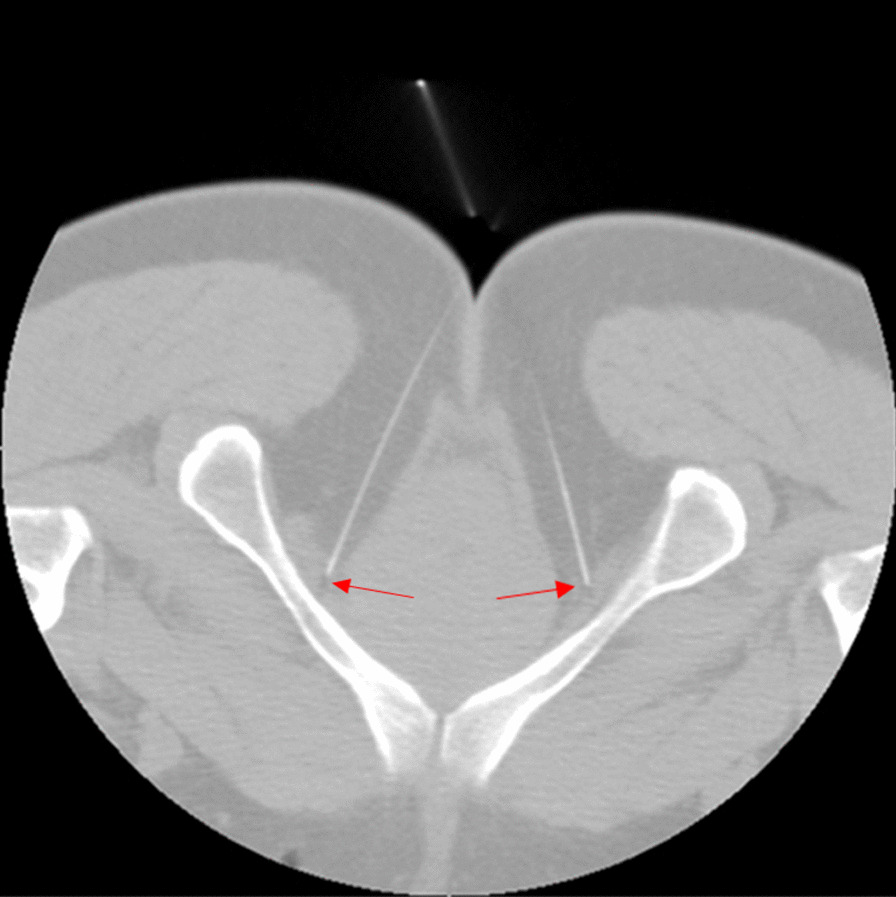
Transverse computed tomography (CT) scan of the coccygeal apex: A transverse CT image of the coccygeal apex shows the tips of needles inserted at the lower sacral point in the ischiorectal fossa (adjacent to the pudendal nerve in Alcock’s canal)
